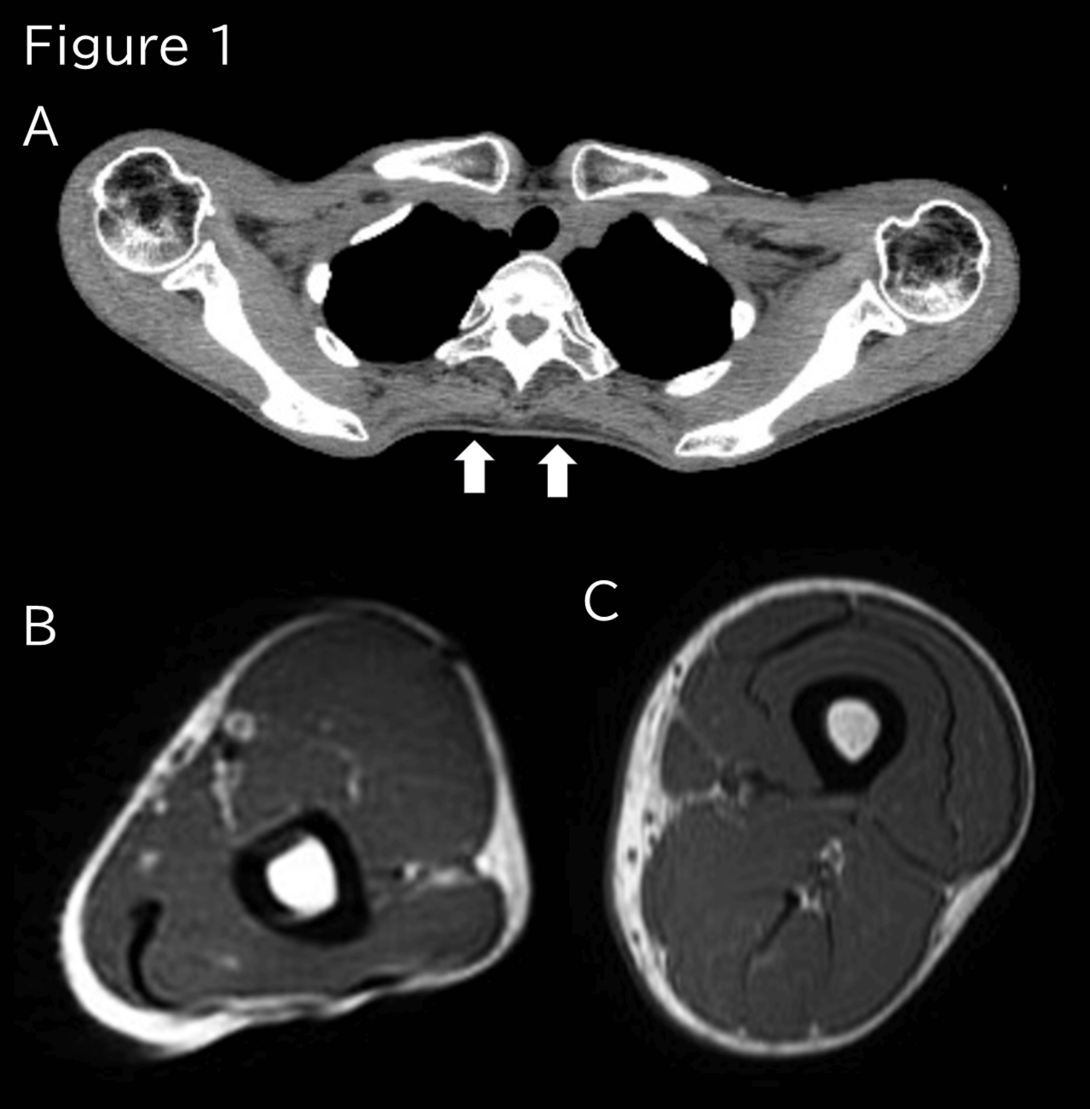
X-ray CT and MRI findings of skeletal muscles. X-ray CT provided evidence of truncal muscle atrophy (A, arrow). T2-WI of the upper (B) and lower limbs (C) showed no atrophy. T2-WI; T2 weighted magnetic resonance imaging
